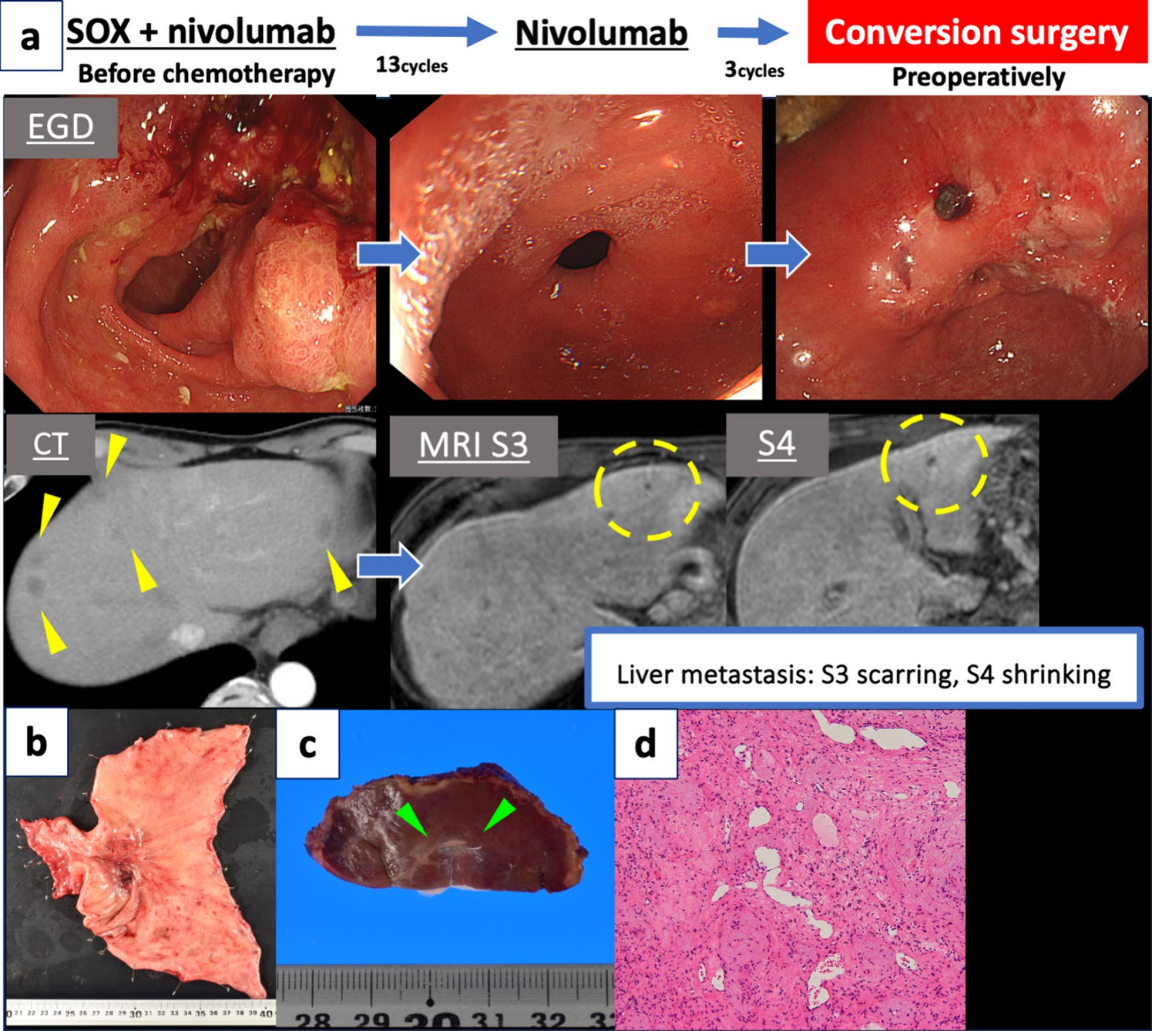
Case 1. a Treatment course in Case 1. The patient was diagnosed with advanced gastric cancer and multiple liver metastases (yellow arrowheads) and received SOX plus nivolumab therapy. After 13 cycles of chemotherapy, all liver metastases except those in S3 and S4 were resolved, and the primary lesion was remarkably reduced. MRI showed two remaining liver metastases measuring 5 mm in segment 4 and 3 mm in segment 3 (yellow dashed lines). The patient developed sinus occlusion syndrome caused by oxaliplatin and received three additional cycles of nivolumab monotherapy. The primary lesion slightly increased in size, but the liver metastases remained at a reduced size. The patient thereafter underwent conversion surgery. b A Borrmann type 3 tumor was found in the gastric antecubital area, and the pyloric ring was deformed. c A white scar was visible on the surface (green arrowheads). d The resected liver tissue had transformed into fibrous tissue devoid of malignancies. *EGD* esophagogastroduodenoscopy, *CT* computed tomography, *MRI* magnetic resonance imaging
